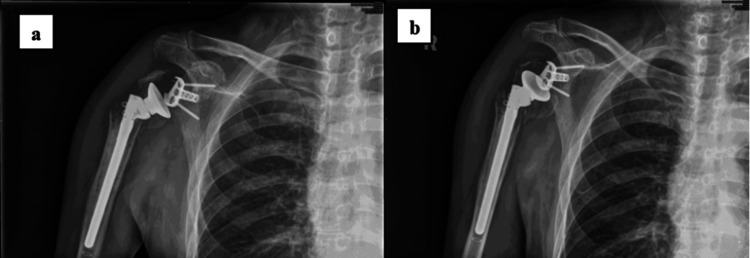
Radiographs of the right shoulder taken three months after reverse shoulder arthroplasty show callus formation in the tuberosities with no evidence of prosthesis loosening. (a) Anteroposterior view; (b) Lateral view
